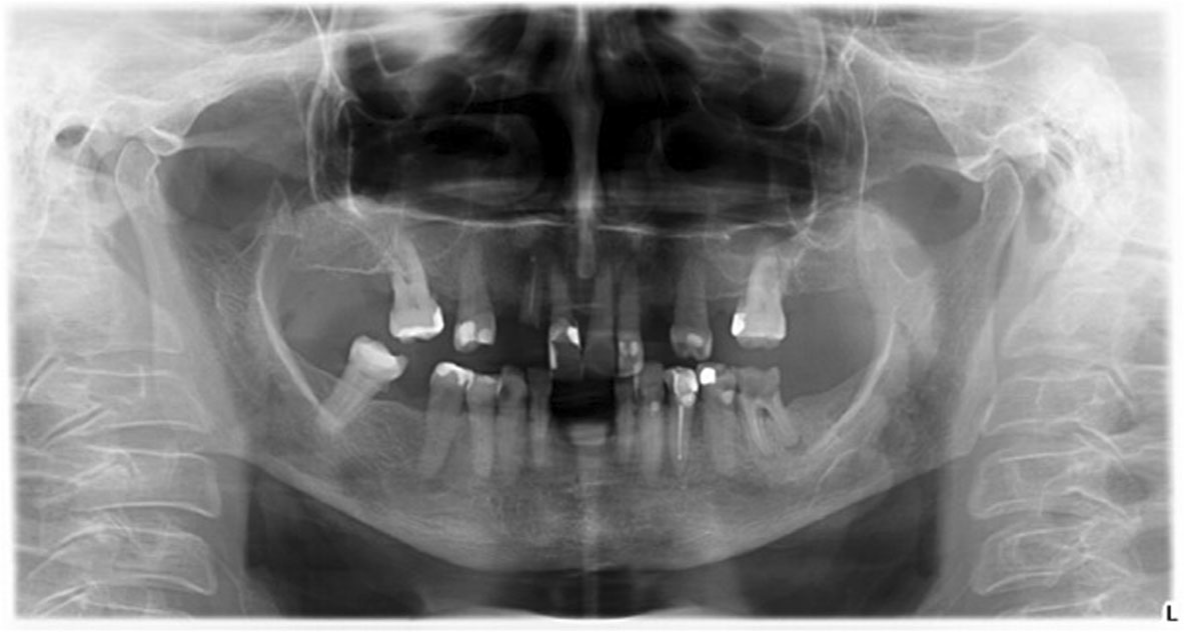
Panoramic radiograph with C3 category of MCI
